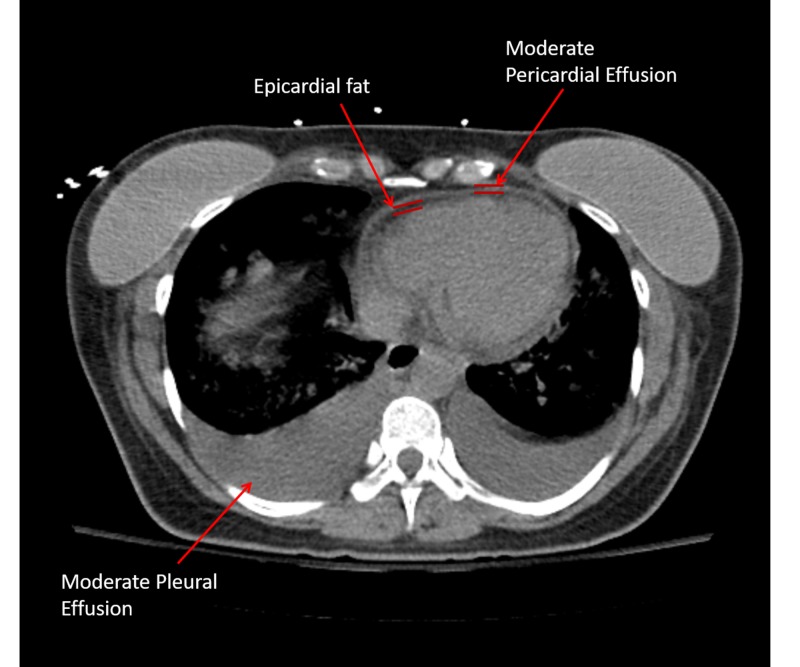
Computed tomography (CT) chest showing pericardial effusion. Computed tomography demonstrated a moderate pericardial effusion in a patient with a normal heart size, moderate pleural effusions and marked peribronchovascular ground glass opacifications (representative of pulmonary edema).
